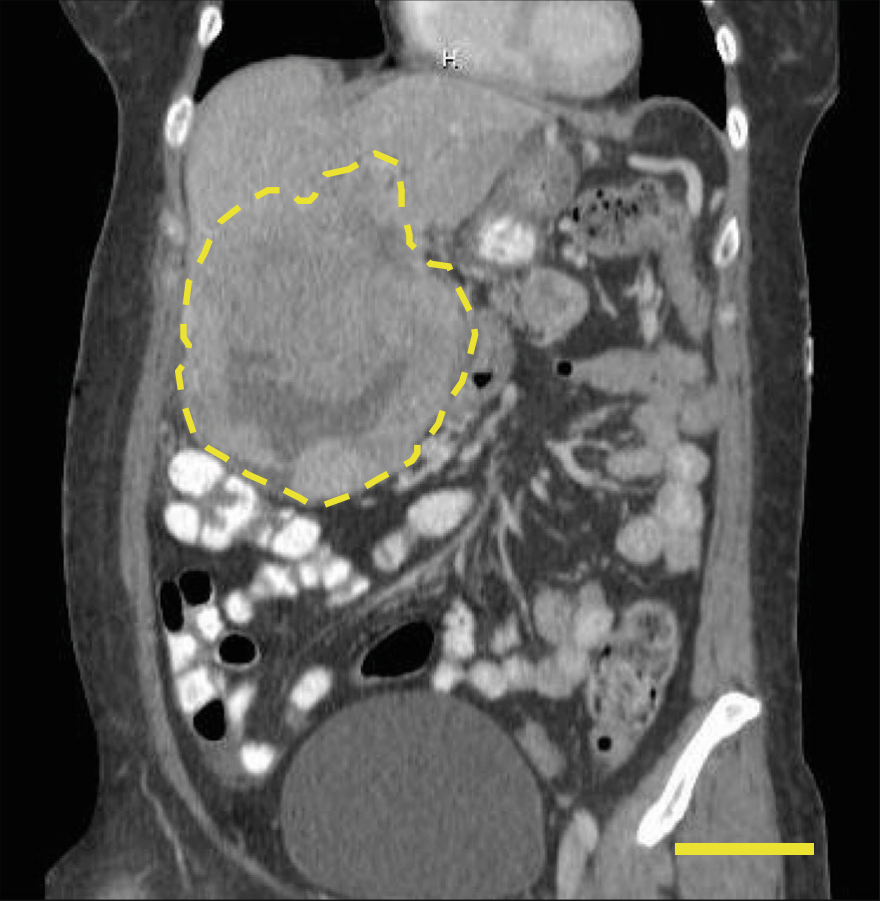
CT scan of causative upper right quadrant mass (indicated with dashed line). Scale = 5 cm
